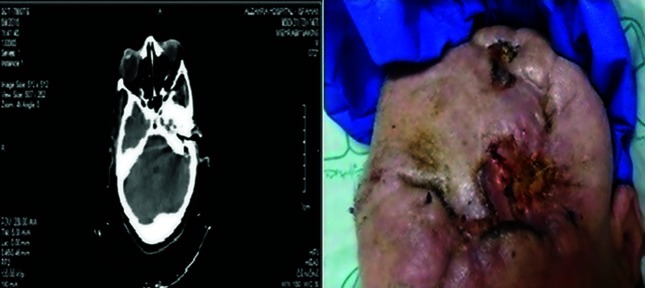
CT scan (left window) and the surgical site on the patient’s head (right window).
